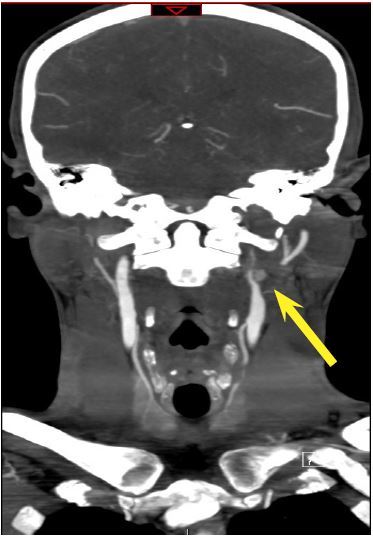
Computerized tomography angiography (CTA) coronal view demonstrating the dissection with the pseudoaneurysm (yellow arrow).
